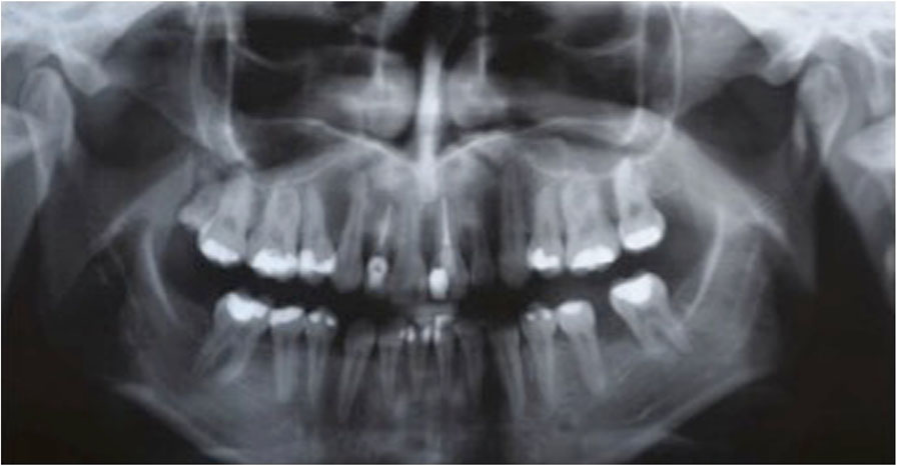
Panoramic radiograph pre-treatment endodontic
